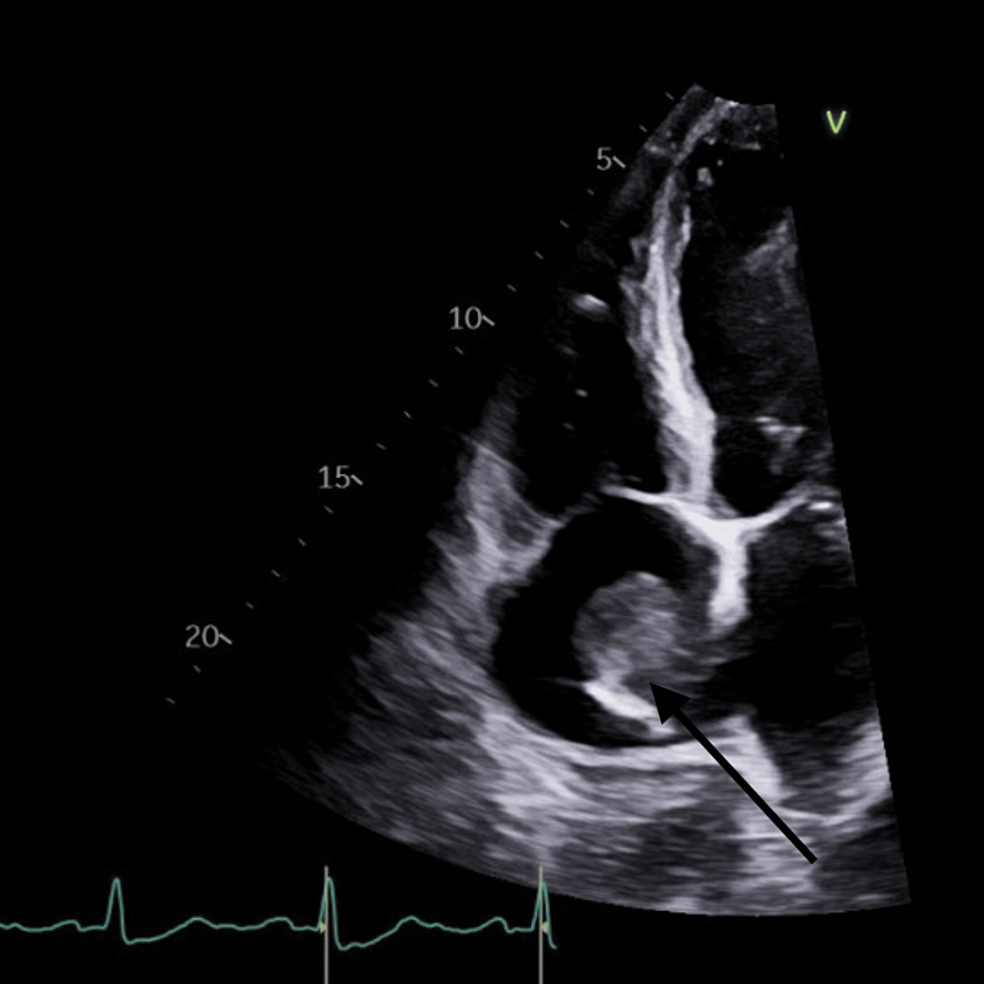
Right atrial mass Right atrial mass seen on a transthoracic echo
